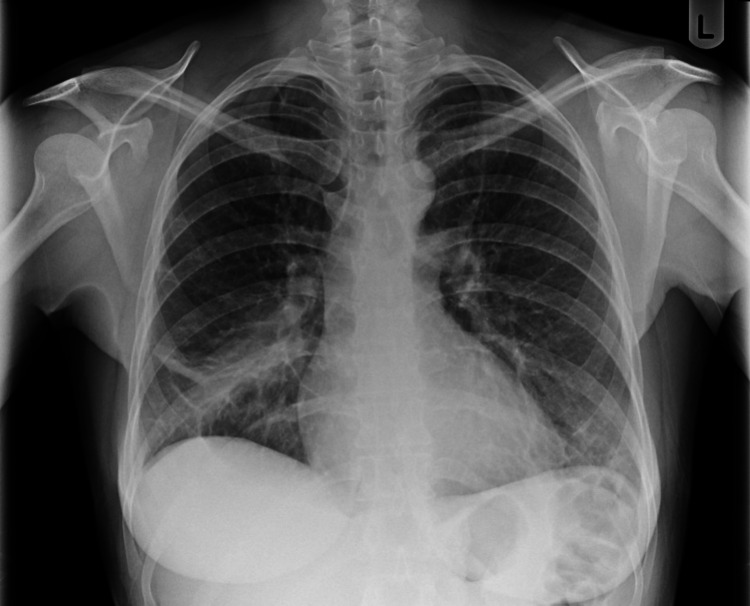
Chest X-ray one month after discharge. Chest X-ray taken one month after discharge from hospital demonstrating only partial improvement in consolidation.
